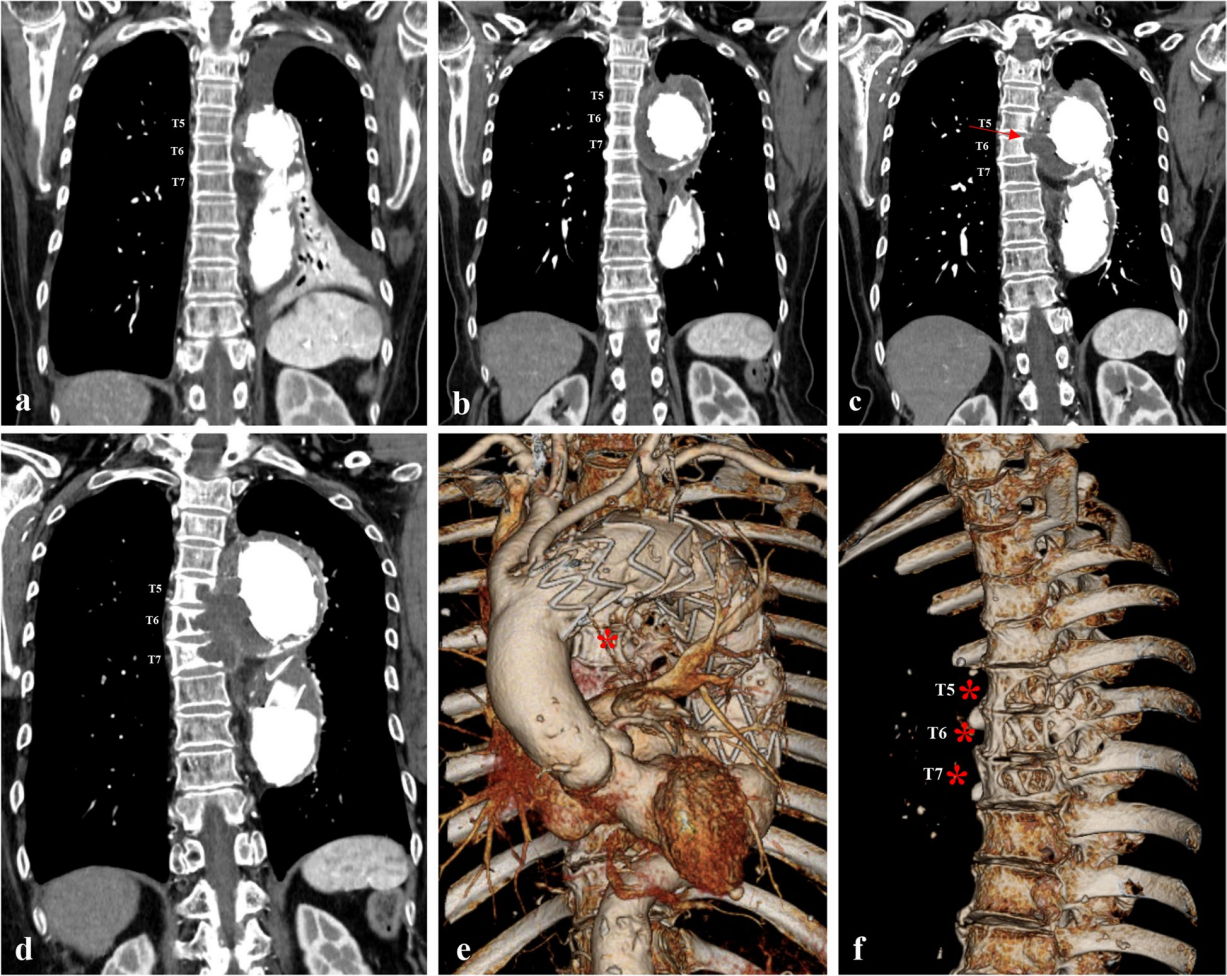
Progression of the thoracic vertebral body erosion in this case. a, Immediately after thoracic endovascular aortic repair (TEVAR), there was no contact between the thoracic aorta lesion and the thoracic vertebral body. b, At a 1.5-year follow-up visit after TEVAR, computed tomography (CT) revealed an increased size of the descending aorta, which was in contact with the thoracic vertebral body. c, At a 3-year follow-up visit after TEVAR, CT showed the perianeurysmal outpouching lesion involving T5 and T6. d–f, At a 4-year follow-up visit after TEVAR, CT revealed a more aggravated perianeurysmal outpouching lesion, and three-dimensional reconstruction of CT data revealed that an anterolateral portion of the thoracic vertebra body had eroded at T5–T7
